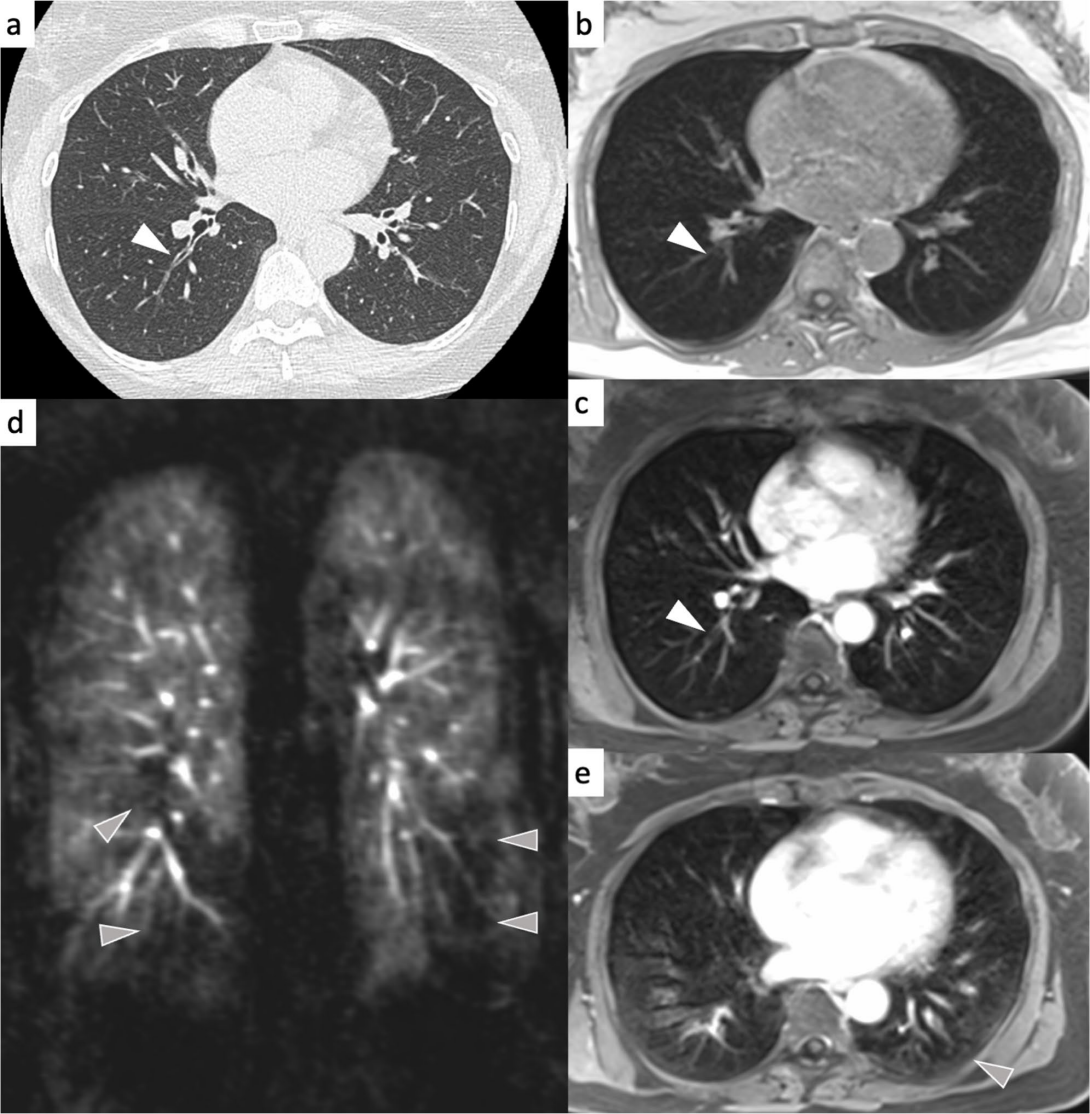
Airway-type COPD in a 57-year-old female participant (classified as airway predominant COPD phenotype). The white arrowhead on the CT image (a) indicates thickened bronchial walls in the right lower lobe. On the corresponding MRI, bronchial wall thickening appears less conspicuous with a slightly better visualisation on the contrast-enhanced transverse 3D gradient echo image (c) compared to the non-contrast-enhanced (b, white arrowheads). Functional deficits from airway disease appear as perfusion defects on the DCE series (d, grey arrowheads) and as air trapping on the contrast-enhanced transverse 3D gradient echo image in expiration (e)
